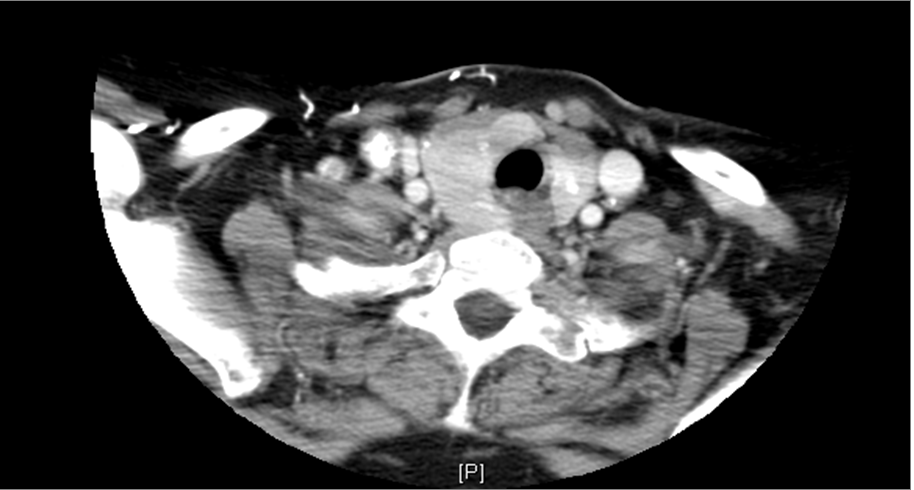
A neck CT scan with contrast of the patient showing a right thyroid lesion.
